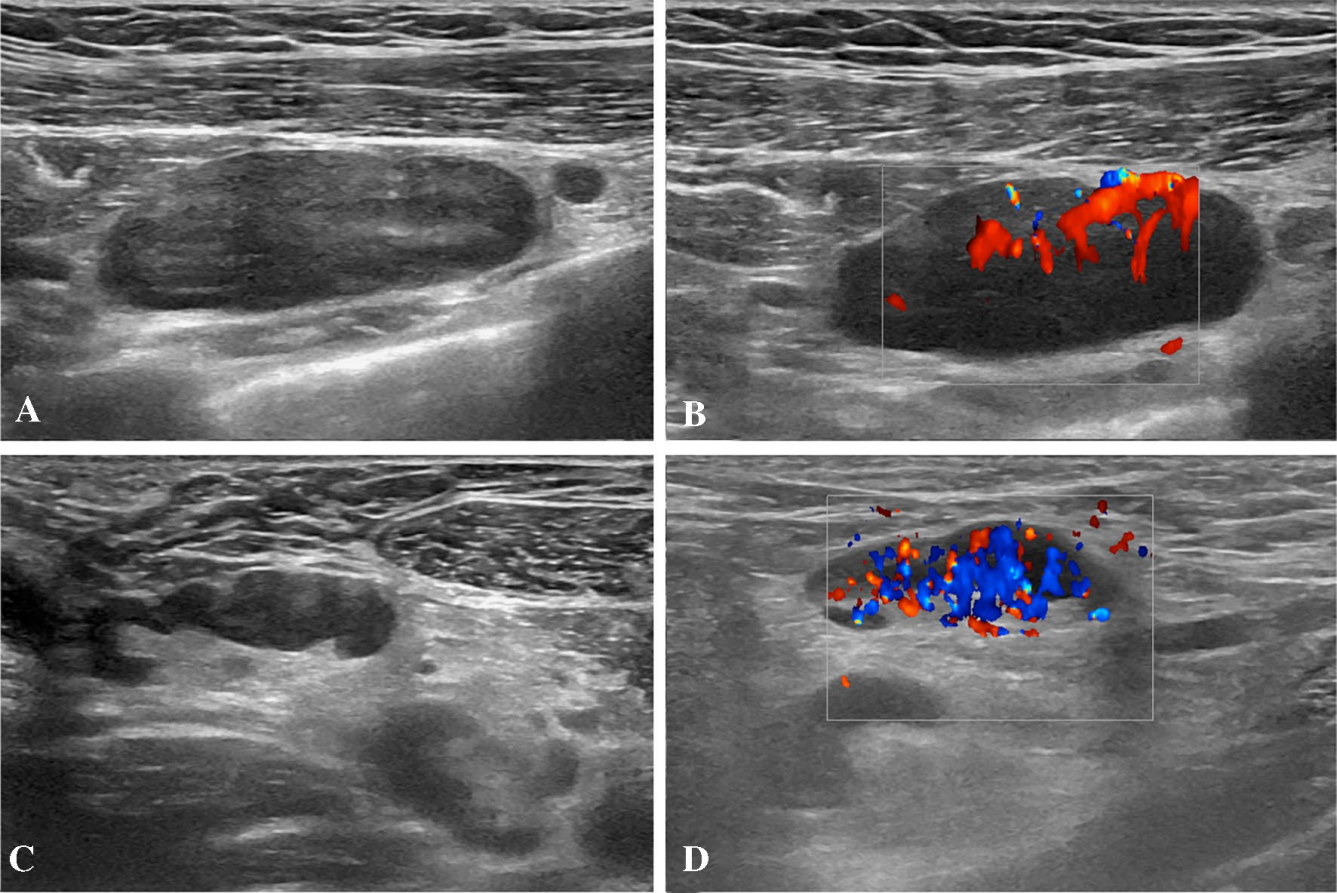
B-Mode (A, B) and color-Doppler (B, D) US examination performed in a 28-year-old woman 2 days after receiving the second dose of vaccine. Enlarged (33 × 11 mm) subclavicular lymph node with no detectable fatty hilum (A) and vivid hilar and cortical vascularization (B) was detected. Enlarged axillary lymph node (26 × 7 mm) with cortical thickening (5 mm) (C) and increased vascularization (D) were also found
